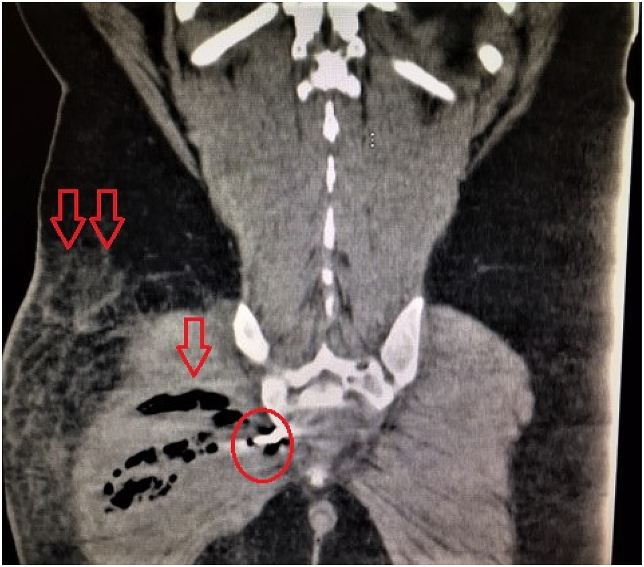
CT coronal view showing the screwdriver end (circled in red) piercing through gluteus muscle with intramuscular (arrow) and subcutaneous emphysema (double arrows).
